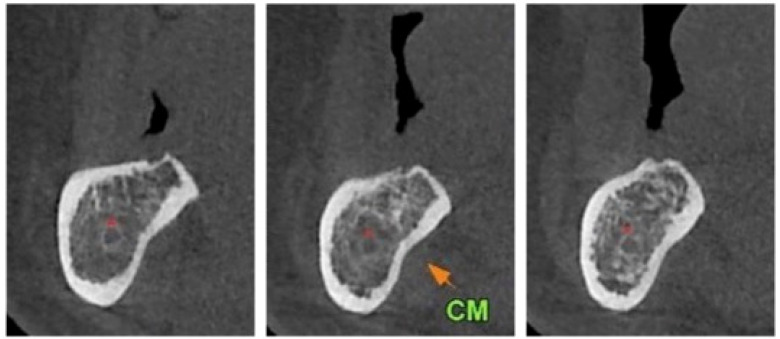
Preoperative Cone Beam Computerized Tomography (CBCT) showing the vertical bone atrophy.
